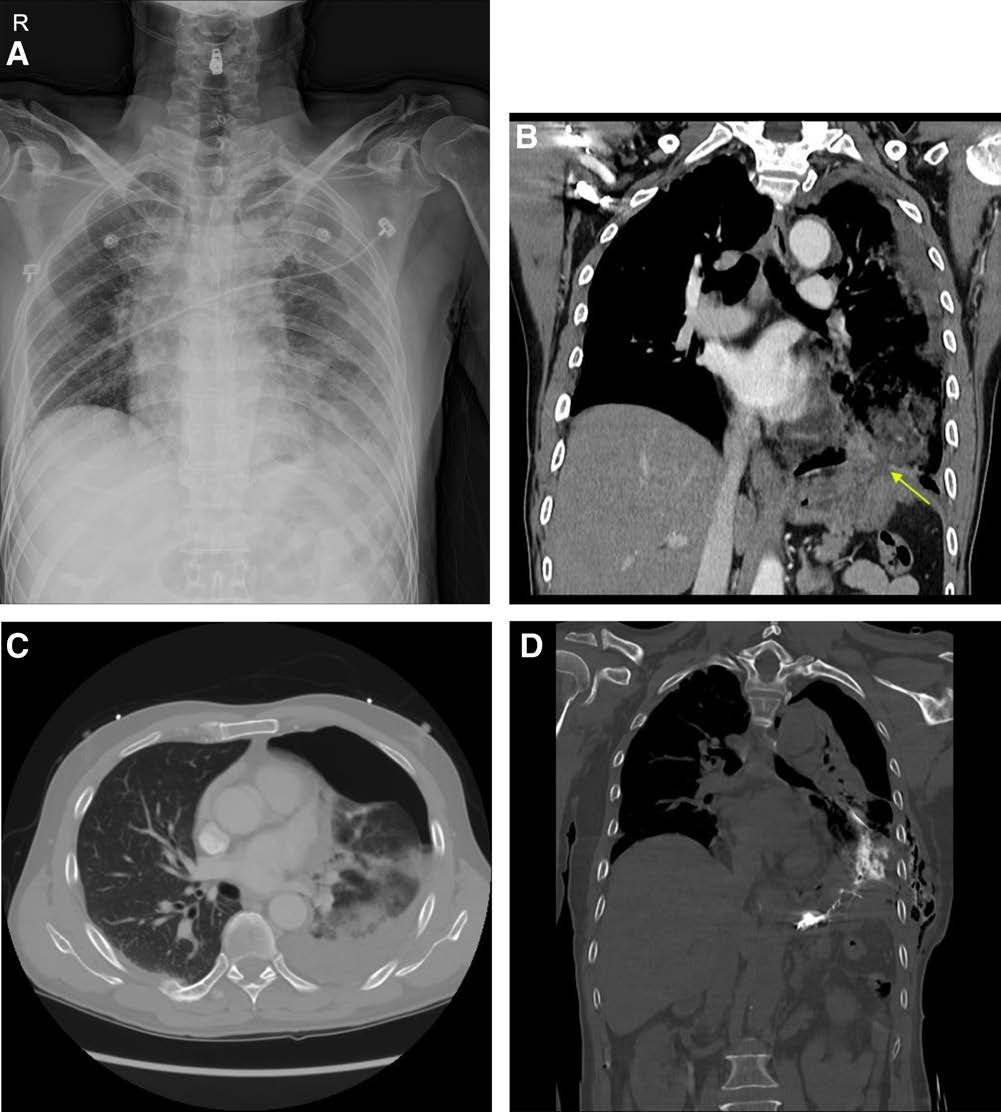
(A) Initial chest radiography. (B) Coronal view of contrast-enhanced chest computed tomography scan. Gastric, diaphragmatic rupture and communication with pleural space were suspected (yellow arrow). (C) Axial view of contrast-enhanced chest computed tomography scan. Left hydropneumothorax with pneumonia. (D) Noncontrast-enhanced chest computed tomography scan after oral contrast swallowing. Oral contrast was observed in the stomach and lung parenchyma.
